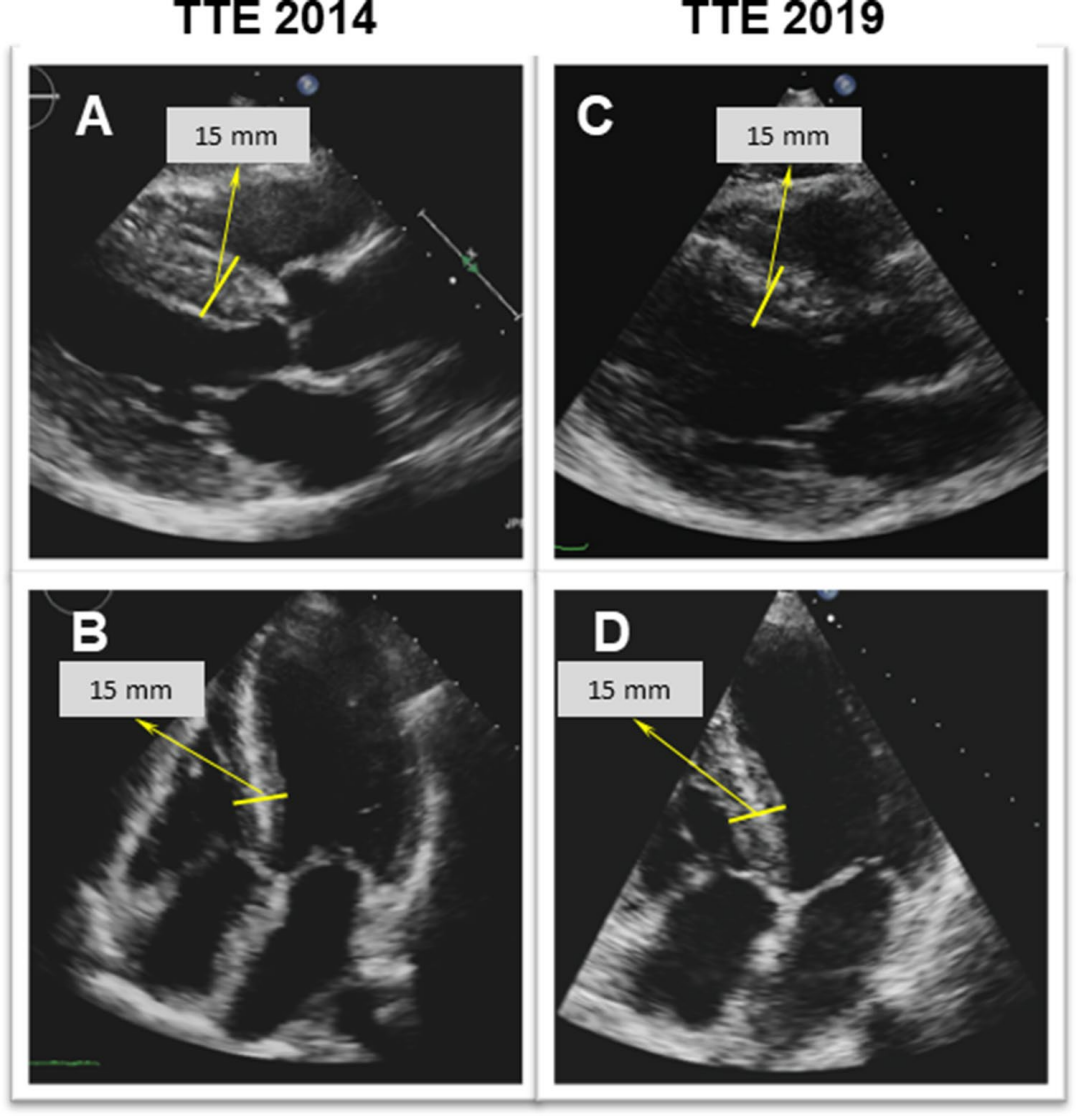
Two-dimensional transthoracic echocardiography still frames at end-diastole in parasternal long-axis (a, c) and apical four-chamber (b, d) views from year 2014 (around the first CMR examination; a, b) and at present evaluation (2019; c, d). Concentric LV hypertrophy can be seen at both time points—without any relevant progression from 2014 to 2019
